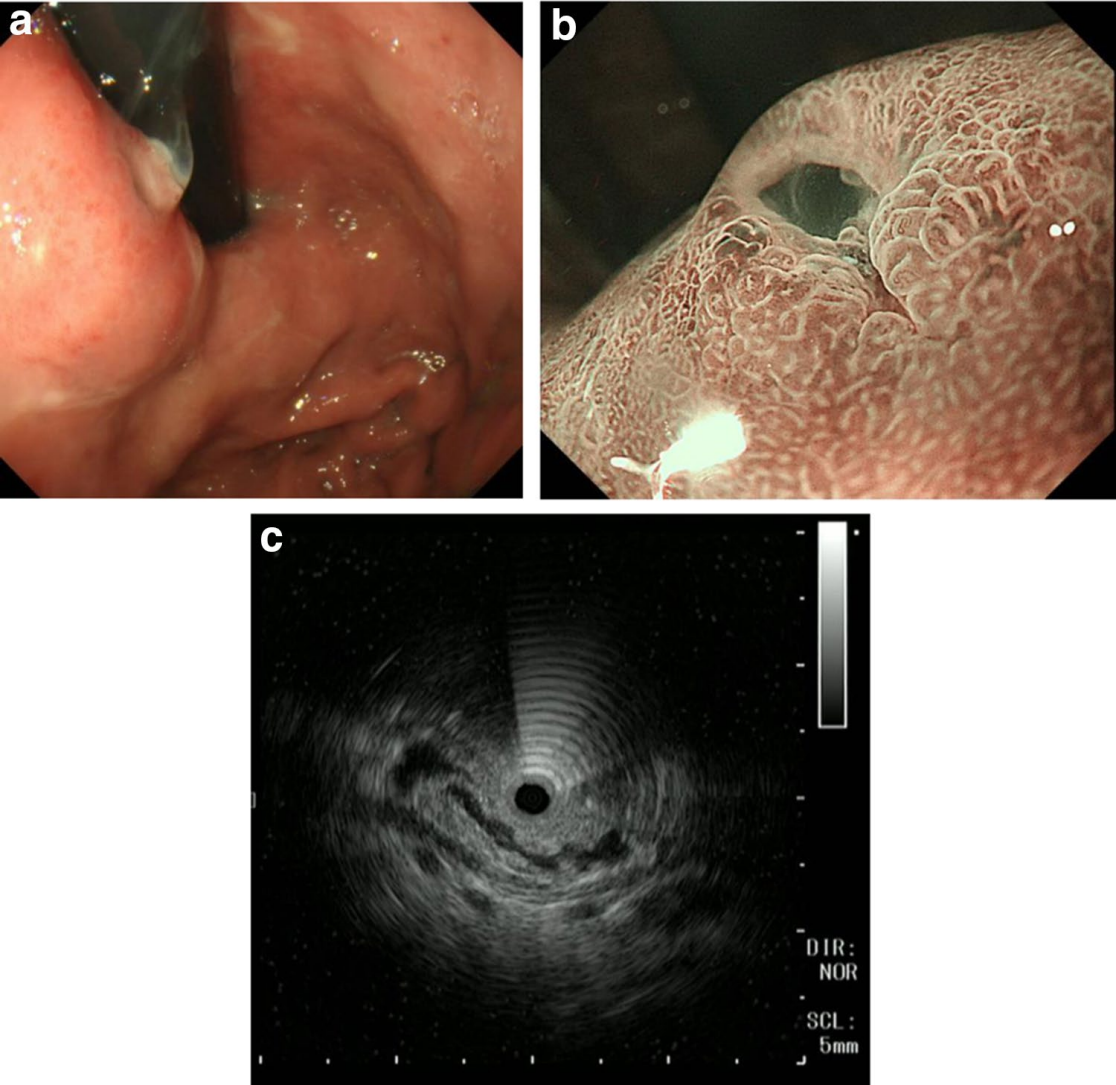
a A 25-mm submucosal tumor-like protrusion shows a slit-like opening at the top. b Dilation of the intervening portion between the crypts is observed on magnifying endoscopy with a narrow-band imaging. Regular surface and microvascular patterns without a demarcation line are noted. c With an endoscopic ultrasonography, low echoic lesion is observed in the third layer; however, the fourth layer remains intact
